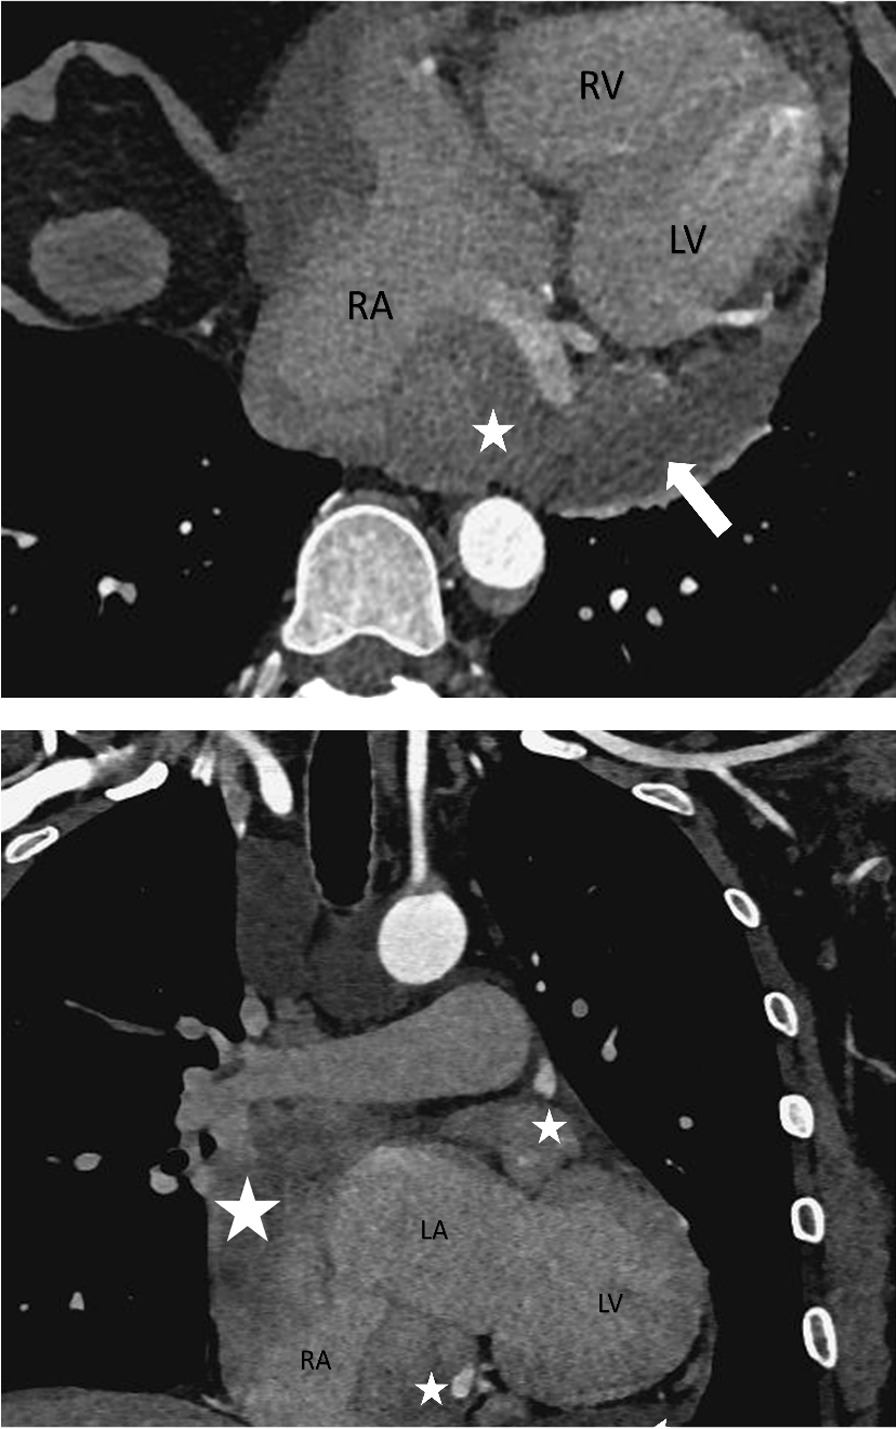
Both the axial and coronal CT images demonstrated anomalous soft tissue masses (pentagon) present in the right atrium, diffusely infiltrating and penetrating the atrial wall, resulting in the disappearance of the normal structure of the right atrium. Visible pericardial effusion (white arrow) was observed surrounding the heart. Abnormal nodules (pentagon) were observed around the left anterior descending coronary artery on the coronal image. *LA* Left atrium, *RA* Right atrium, *LV* Left ventricle
